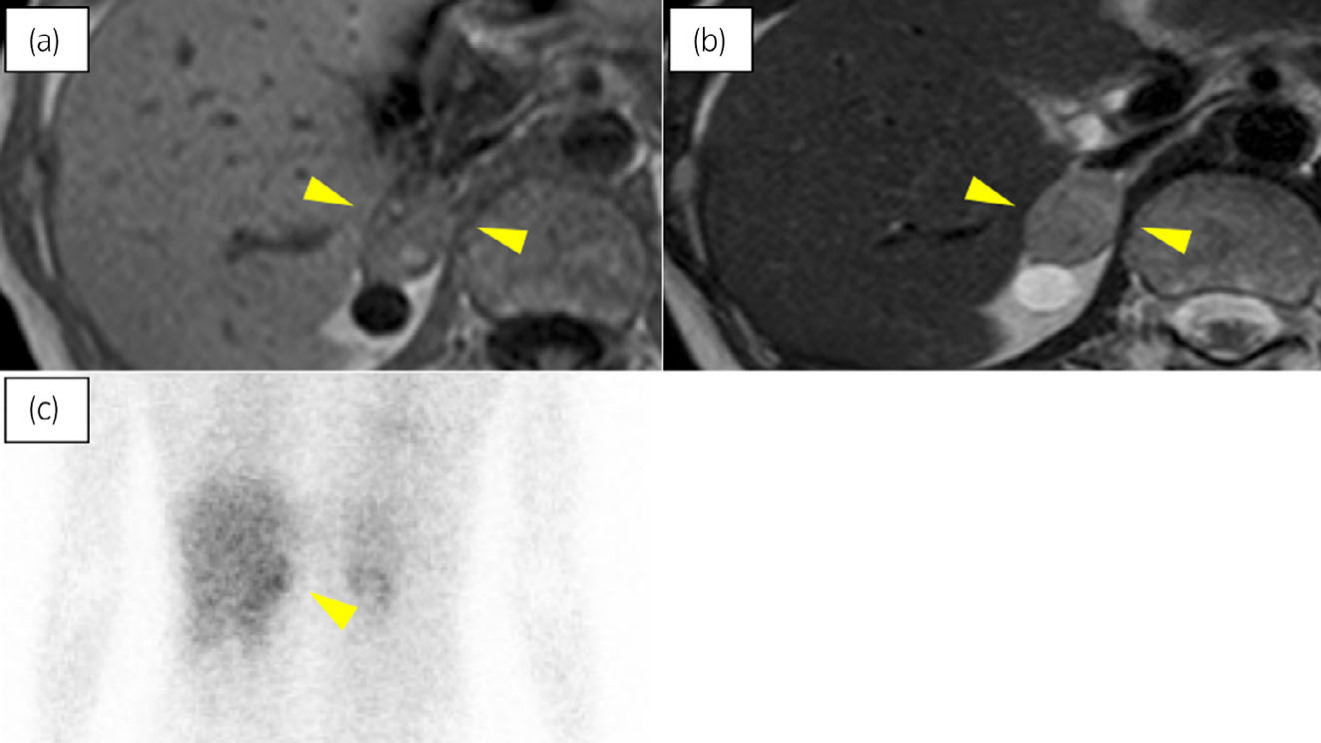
Images of the pheochromocytoma. (a) The right adrenal tumor on T1‐weighted MRI, (b) the right adrenal tumor on T2‐weighted MRI, (c) ^123^I‐MIBG scintigraphy showing high accumulation in the right adrenal gland.
